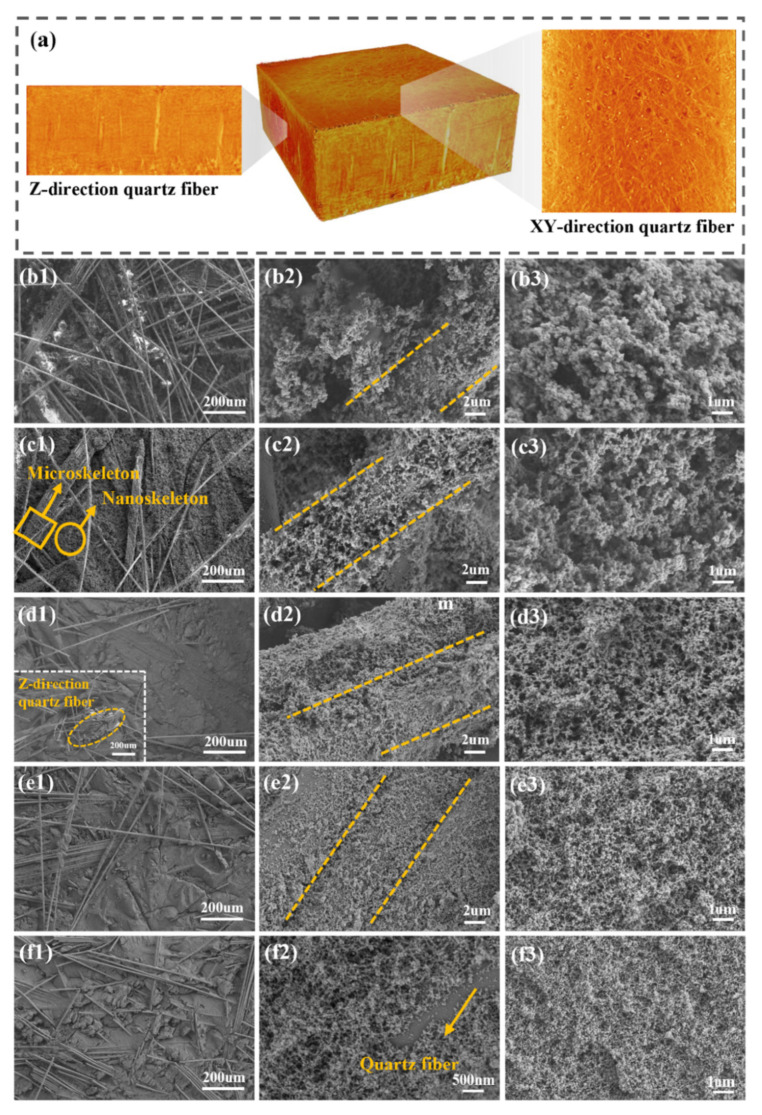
Microstructural morphology of NQF/PR. (a) Reconstructed 3D-CT images of NQF/PR-25. (b1–b3) NQF/PR-10. (c1–c3) NQF/PR-15. (d1–d3) NQF/PR-20. (e1–e3) NQF/PR-25. (f1–f3) NQF/PR-30.
